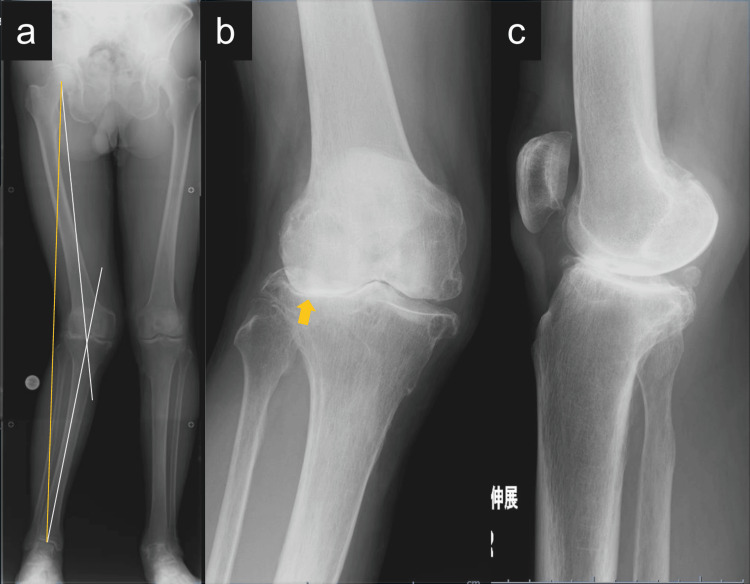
Preoperative radiographs of the right knee. (a) Standing full-length anteroposterior (AP) radiograph of the lower limbs showing severe valgus alignment of the right knee. The hip–knee–ankle angle was −18°, indicated by white lines. The mechanical axis, shown as a yellow line, deviates significantly laterally. (b) AP radiograph of the right knee highlighting joint space narrowing in the lateral compartment, marked by a yellow arrow. (c) Lateral radiograph showing joint line orientation and posterior tibial slope in extension.
